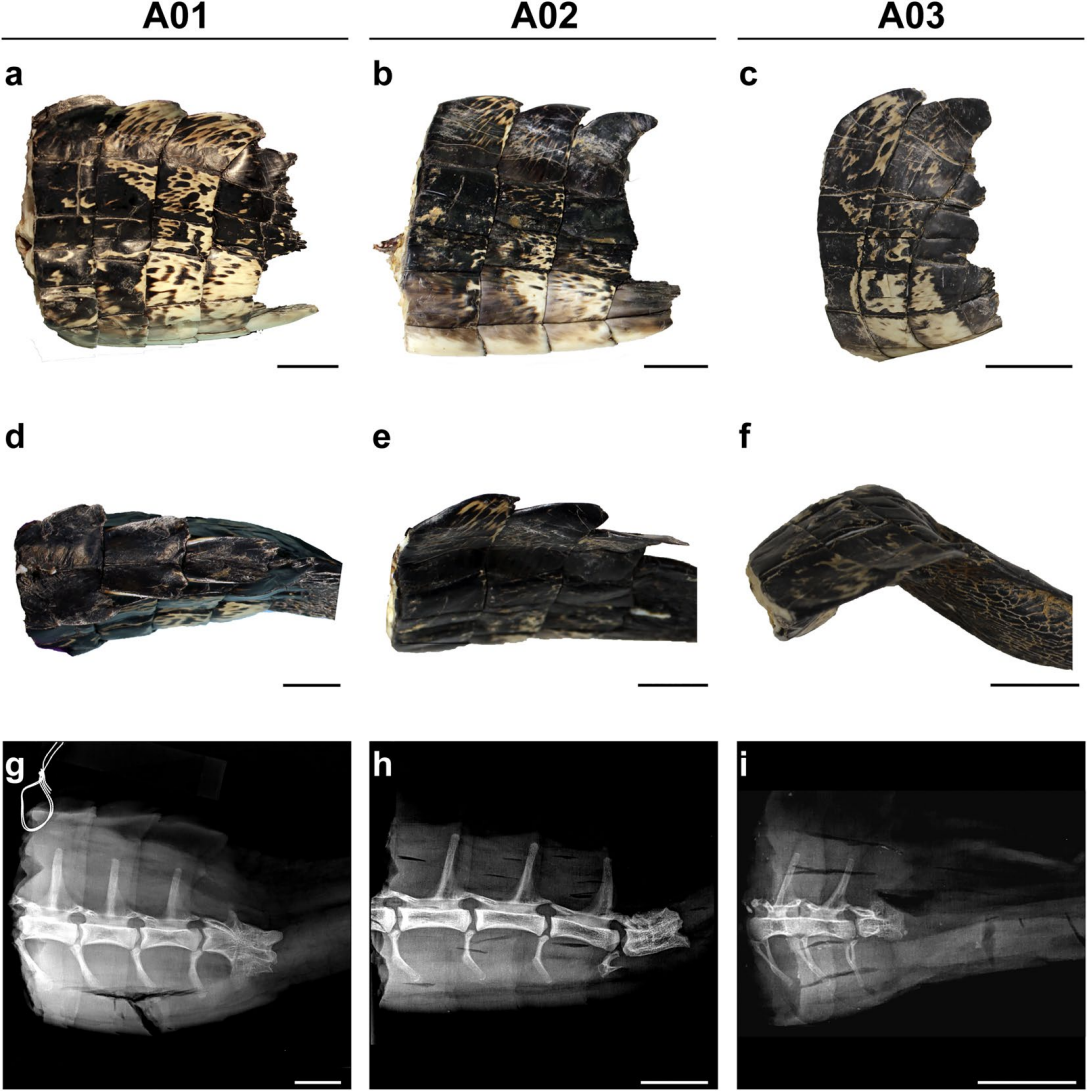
The original tail is covered in organized scales and caudal vertebrae exhibit prominent structures. Lateral views of original tail segments proximal to the site of injury (a–c). Scales are mottled and organized into transverse rows identified by dorsal scutes. Dorsal views of original tails (d–f). A01 exhibits paired dorsal scutes (d) while A02 and A03 exhibit single dorsal scutes (e,f). Lateral radiographs reveal articulated caudal vertebrae that feature prominent neural spines and hypapophyses (g–i). The last remaining caudal vertebra exhibits bone fissures and lacks vertebral processes. Scale bars are 2.5 cm.
